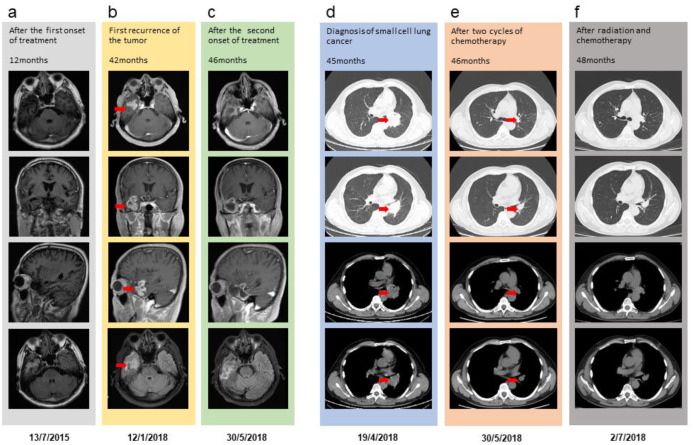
Clinical course of the patient. (a) Twelve months after initial diagnosis, MRI (T1-enhanced, axial/coronal/sagittal; T2-FLAIR axial) showing no evidence of disease. (b) Forty-two months after initial diagnosis, MRI showing recurrent right temporal lobe tumor (red arrow). (c) Forty-six months after initial diagnosis, MRI post-chemoradiotherapy showing stable intracranial disease. (d) Forty-five months after initial diagnosis, chest CT showing left hilar mass (red arrow) and mediastinal lymphadenopathy. (e) Post-chemotherapy (2 cycles) CT showing marked shrinkage of left hilar mass (red arrow). (f) Post-concurrent chemoradiotherapy CT showing stable pulmonary findings. (MRI parameters: 3.0T, slice thickness 5mm; CT parameters: slice thickness 3mm).
